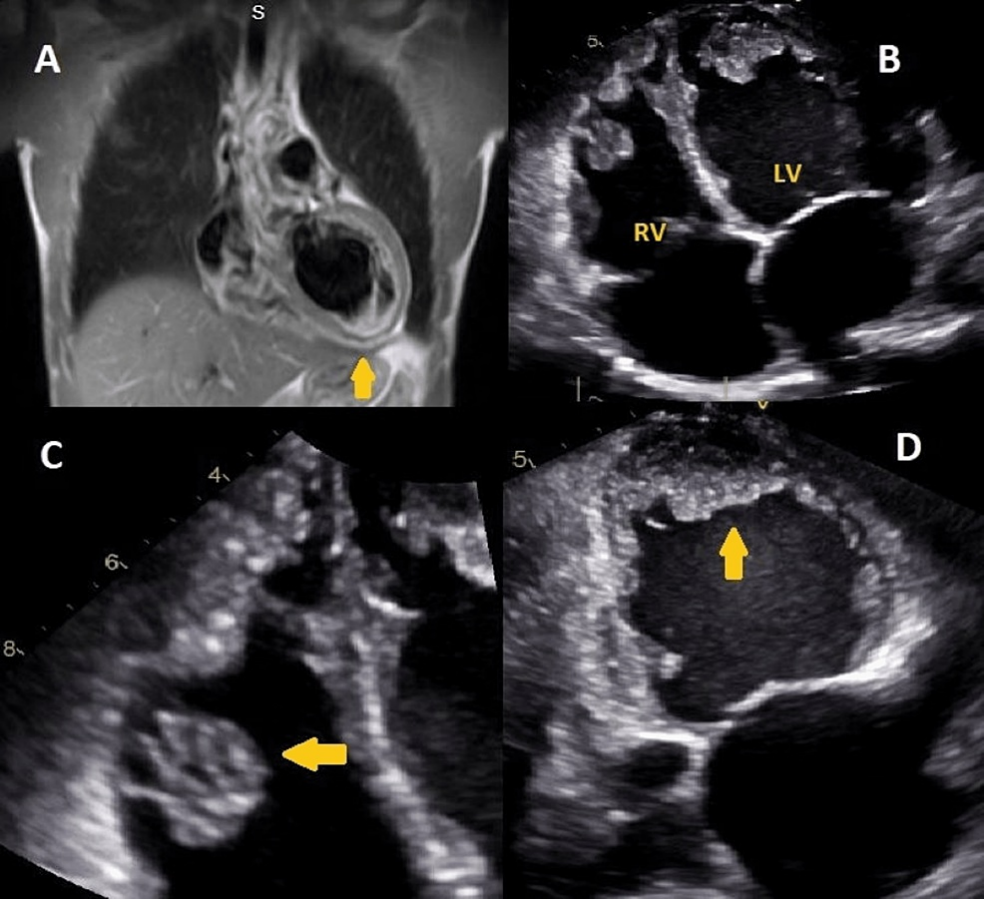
Cardiac MRI with myocarditis findings and echocardiogram done a few months later showing biventricular thrombus (a) Cardiac MRI showing nonspecific mid-myocardial enhancement consistent with myocarditis. (b) Transthoracic echo showing left and right ventricular thrombus along with severe dilation. (c) Right ventricular apical thrombus. (d) Left ventricular mural thrombus MRI: magnetic resonance imaging
